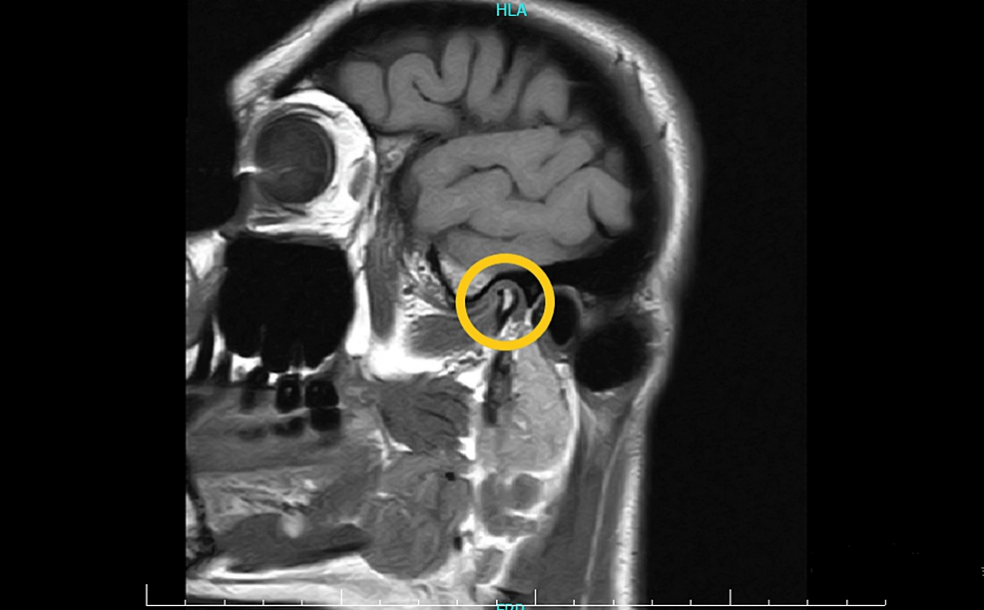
MRI of the patient's right temporomandibular joint before stem cell therapy MRI: Magnetic resonance imaging
